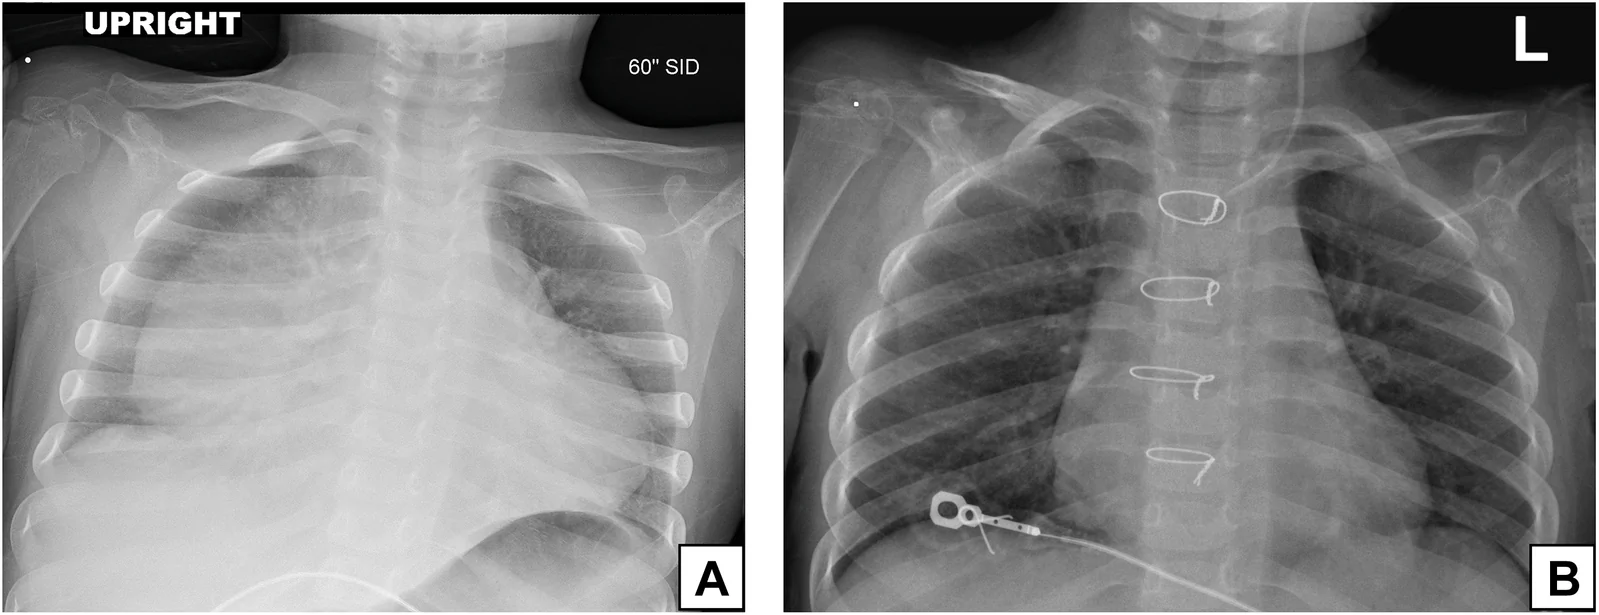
Chest X-Ray Pre- and Post-Atrial Reduction (A) Chest x-ray demonstrating cardiomegaly secondary to right atrial dilation before atrial reduction procedure. (B) Chest x-ray showing normal cardiac border post right atrial reduction procedure.
